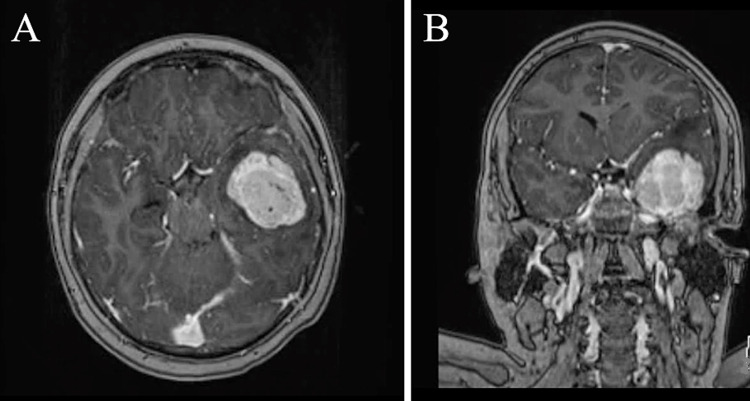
MRI showing an enhancing left temporal tumor T1-weighted axial (A) and coronal (B) MRI showing an enhancing left temporal tumor along the inferior cortical surface with mass effect and significant surrounding vasogenic edema.
